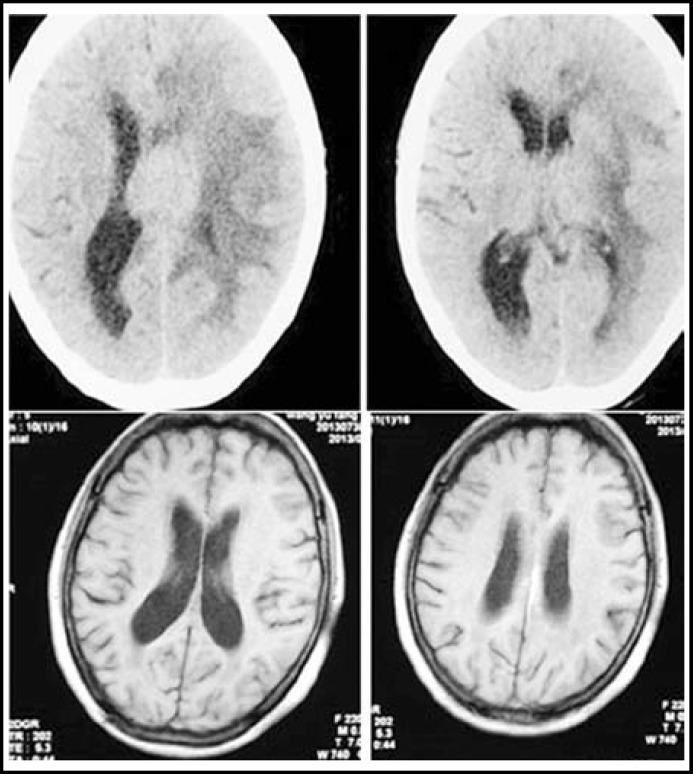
CT examination results before and after clearance of epidural hematoma
